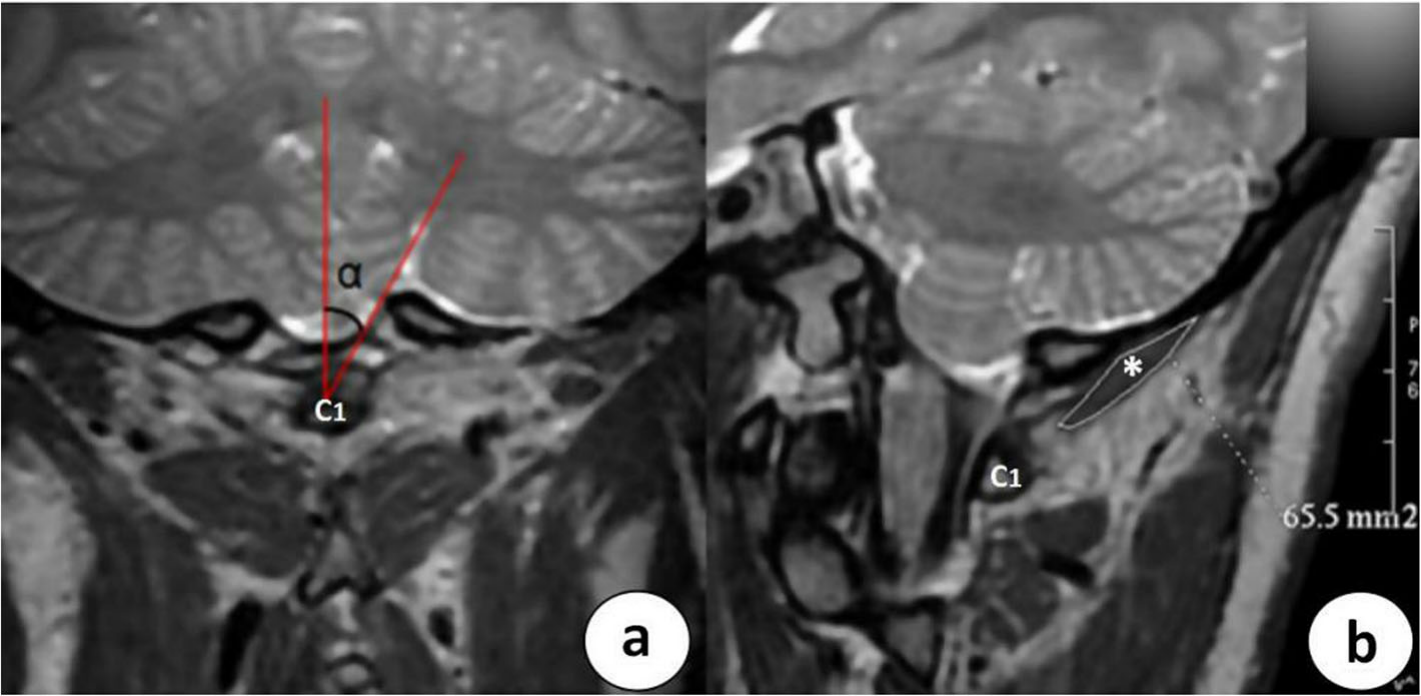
The measurement method for the maximum SA of the RCPmi muscle. a Coronal T_2_-weighted MR image across the posterior arch of the atlas. The SA of the RCPmi muscle is measured on an oblique parasagittal slice with a 30° deviation from the mid-posterior arch of the atlas. b On the oblique sagittal T_2_-weighted MR image defined in a., the SA of the left RCPmi muscle is given by the delineated area (white*) and has a calculated value of 65.5 mm^2^. Note: SA, sectional area; C1: atlas; α = 30°
